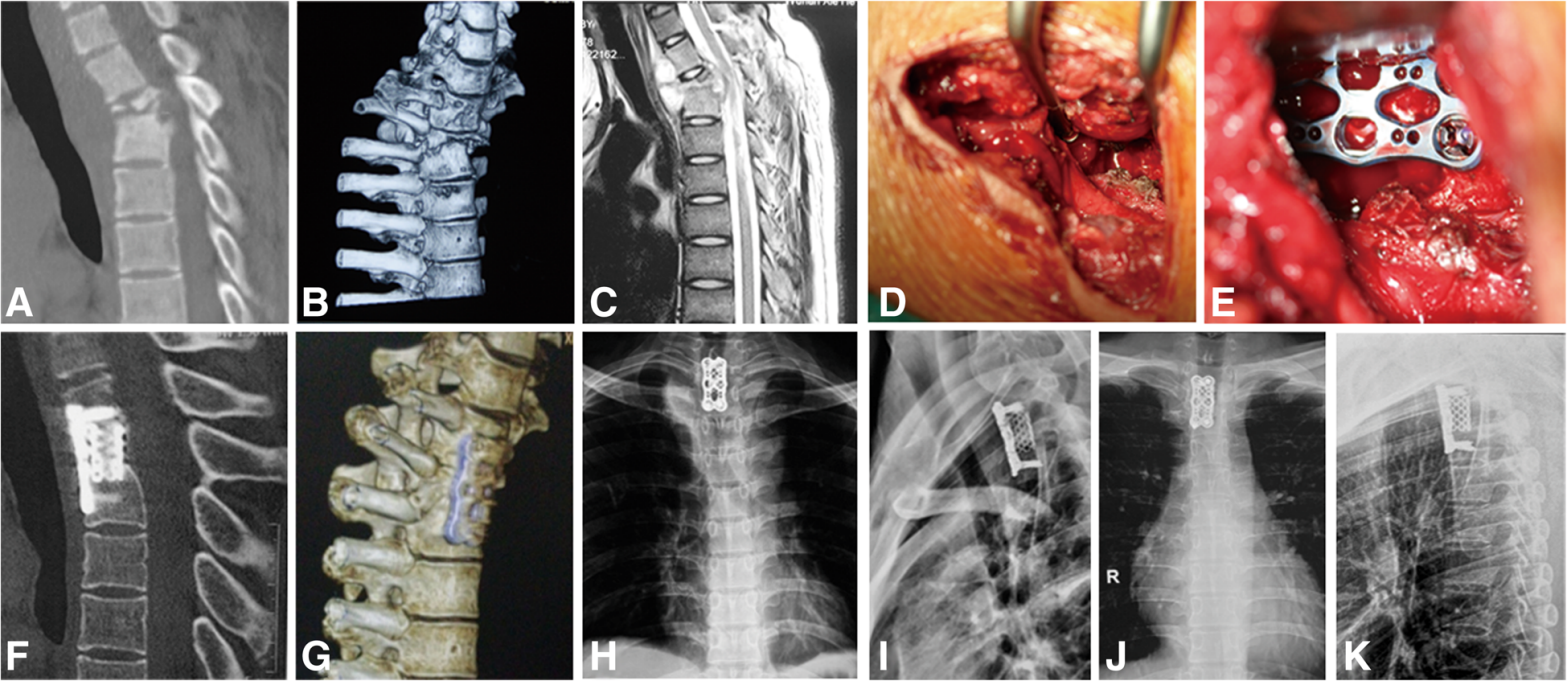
Nineteen-year-old boy with cervicothoracic tuberculosis (T1-3 level). a, b Preoperative computed tomography scan and three-dimensional reconstruction demonstrate vertebral body destruction with kyphosis. c Preoperative magnetic resonance imaging scan. d, e Intraoperative debridement, internal fixation, and fusion with titanium cage strut. f–i Radiographs postoperatively showing well-positioned internal fixation and improved kyphosis. j, k Radiographs showing satisfactory focal clearance and strut graft stability at the final follow-up
